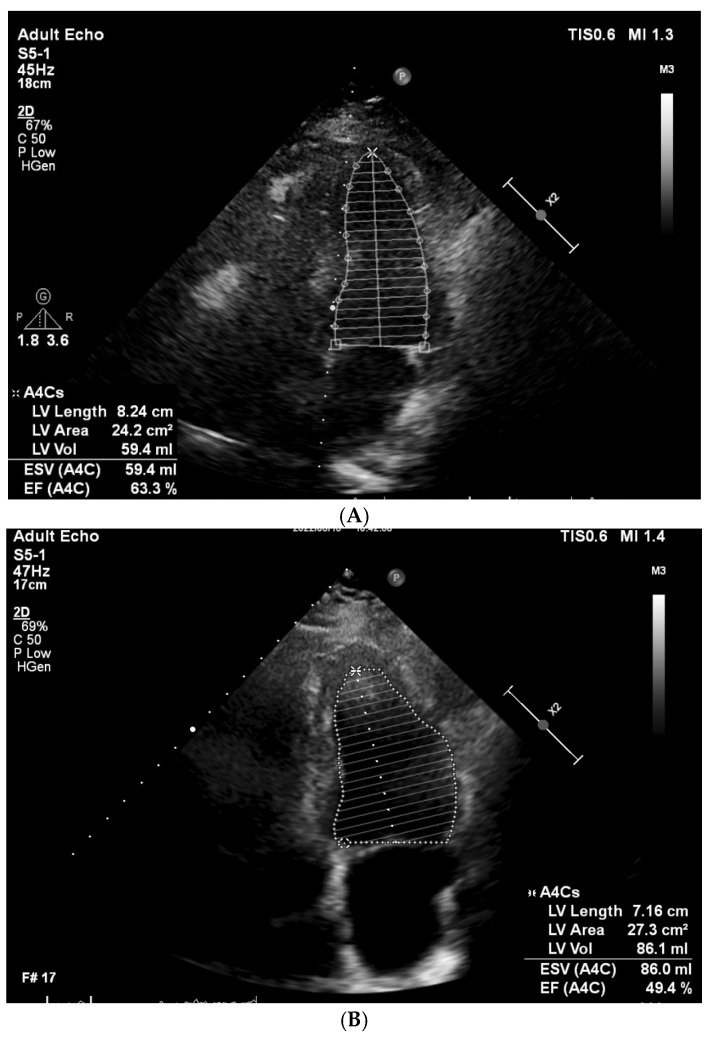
Change in LVEF from 63.3% to 49.4%, calculated using the modified biplane Simpson’s method. (A) baseline echocardiography; (B) follow-up echocardiography 12 months after HSCT. LVEF: left ventricular ejection fraction, HSCT: hematopoietic stem cell transplantation.
